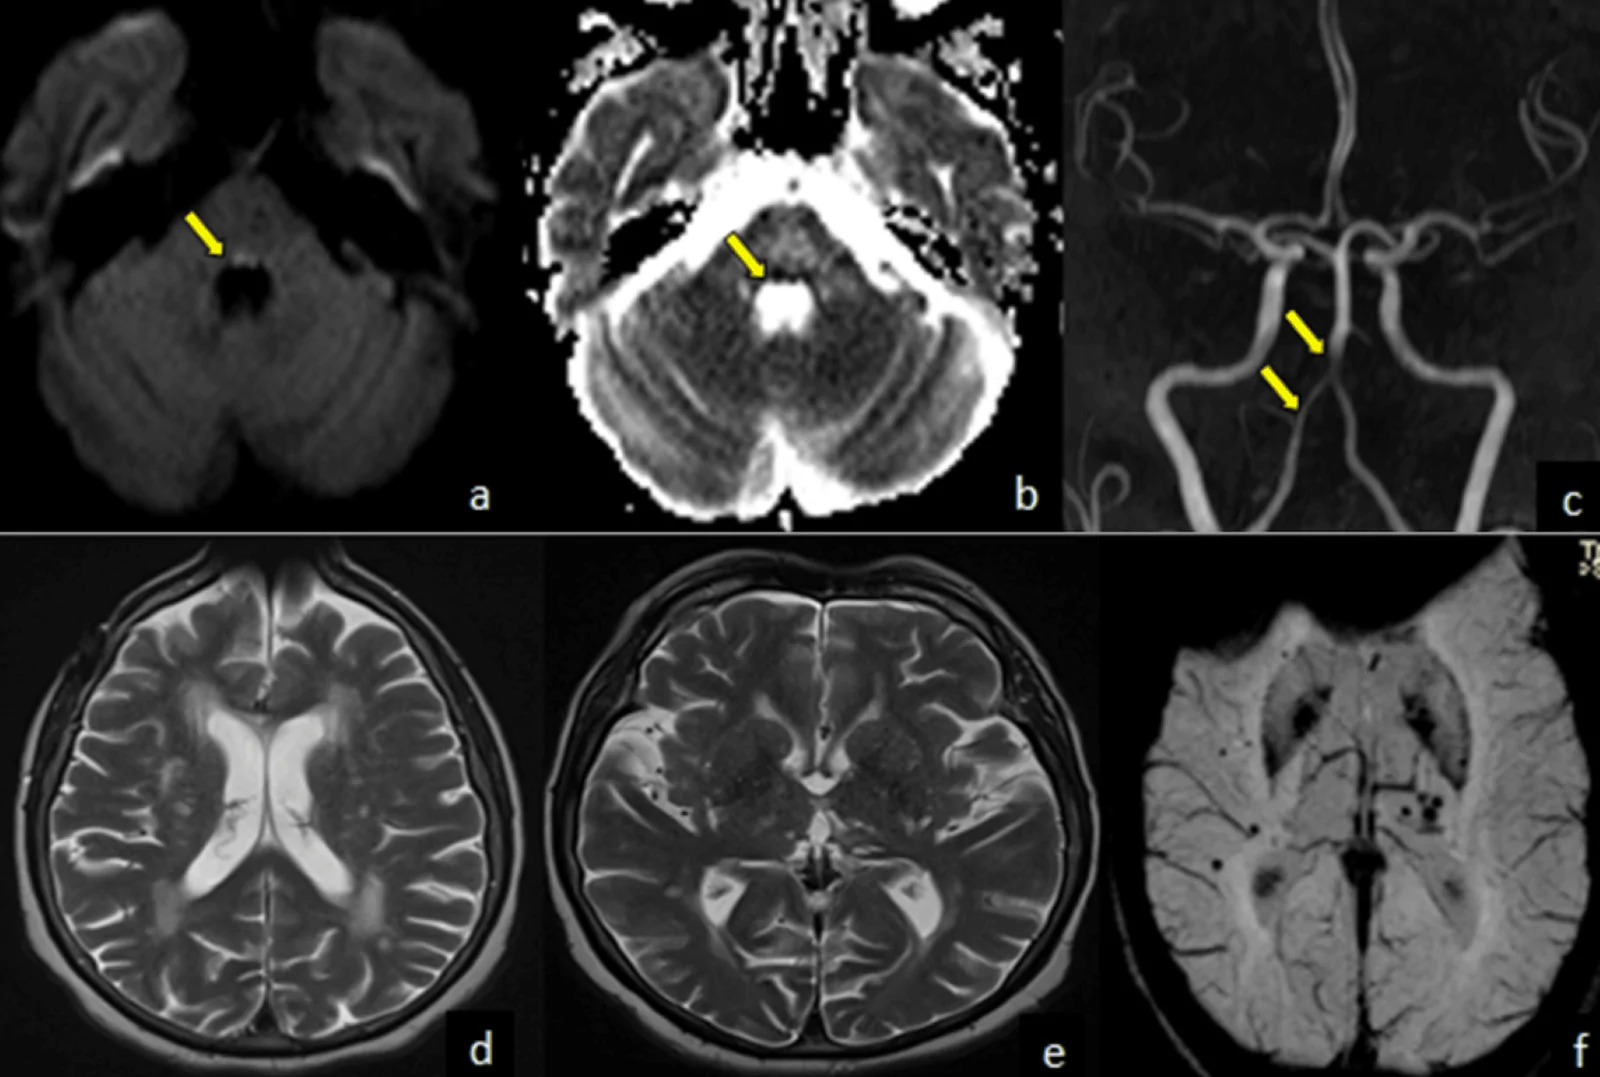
Brain MRI. Diffusion-weighted imaging (DWI) of the brainstem showing two adjacent hyperintense foci within the pontine tegmentum (arrow) (a), with corresponding restricted diffusion on the apparent diffusion coefficient (ADC) (arrow) (b), suggestive of acute bilateral dorsal pontine tegmental infarction. Atheroma in the proximal third of the basilar artery and right vertebral artery on MR angiography (arrows) (c). Vascular leukoencephalopathy (d) and dilated Virchow-Robin spaces (e) on T2-weighted images. Cerebral microbleeds on SWI sequence (f), related to cerebral small vessel disease. SWI: Susceptibility-weighted sequence
